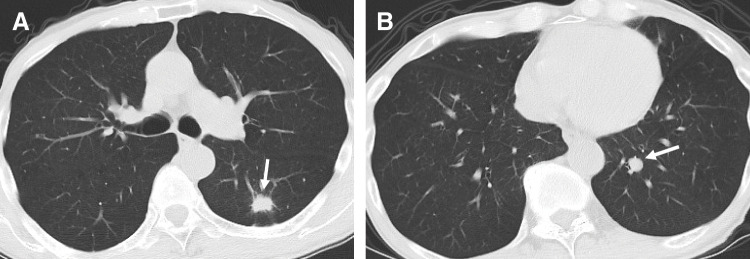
Preoperative chest CT scans. (A) A spiculated nodule measuring 19 × 16 mm was identified in segment 6 of the left lower lobe (arrow). (B) A round nodule measuring 8 × 4 mm was detected in segment 10 (arrow).
